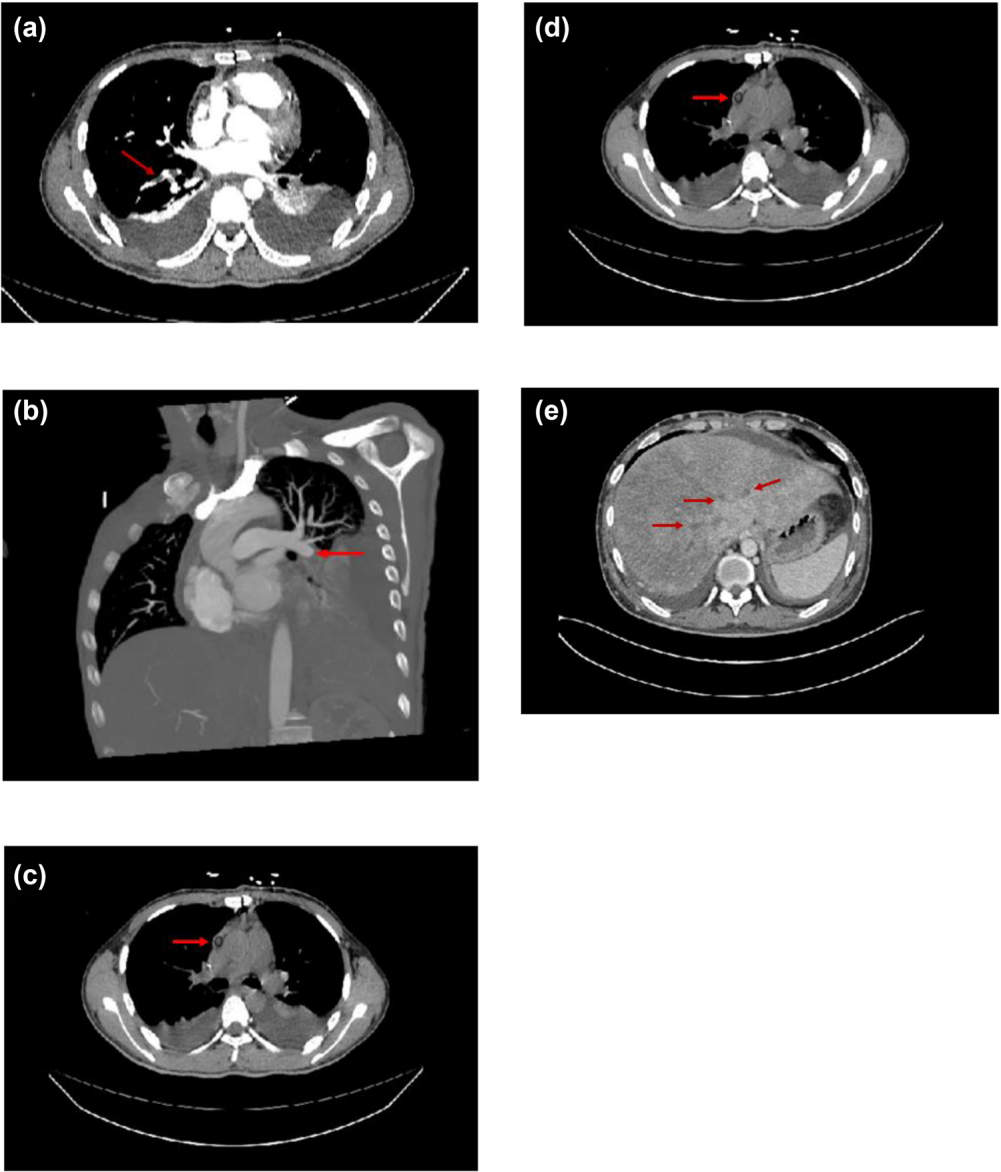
CTA imaging showing vascular filling defects. Note: (a) right pulmonary artery embolism, (b) left pulmonary artery thrombosis, (c) intracardiac thrombosis, (d) inferior vena cava thrombosis, and (e) thrombosis in the left, middle, and right hepatic veins.
